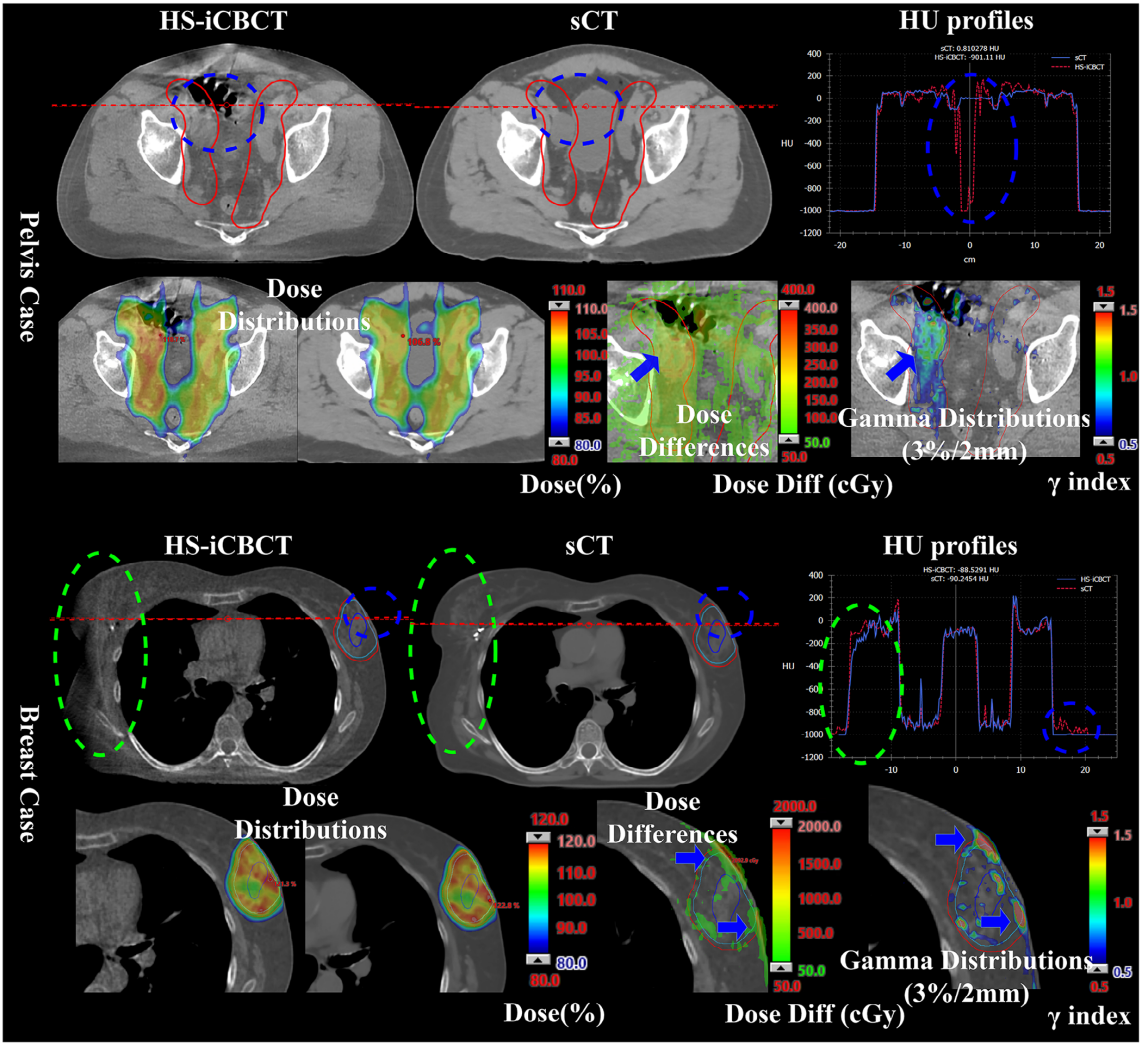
The example slices, HU profiles, dose distributions, dose differences, and gamma distributions for two example cases from pelvis and breast sites. Blue arrows highlight the distortions of sCT and following dosimetric errors. Blue dash circle highlights the failure of sCT to monitor the patient daily anatomical variation and following dosimetric errors. Green dash circles highlight the limited FOV of HS‐iCBCT, noticeable in areas far from the isocenter. FOV, field of view; HU, Hounsfield‐units; HS‐iCBCT, HyperSight iterative CBCT; sCT, synthetic‐CT.
